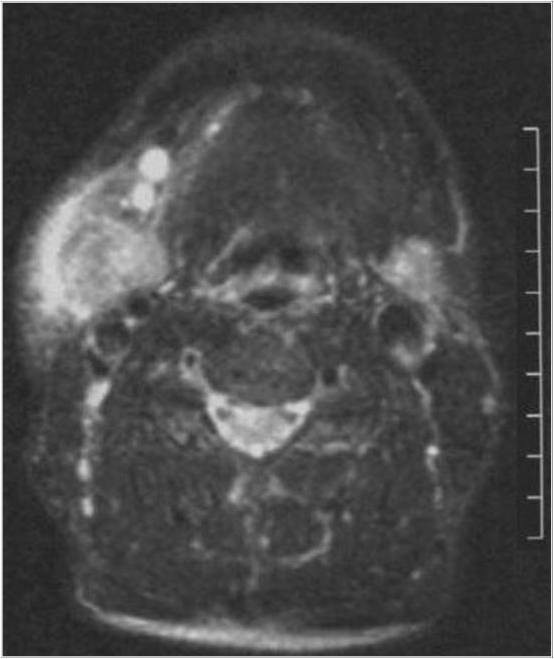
Fat-saturated T2-weighted weighted MRI. Fat-saturated T2-weighted weighted MRI showing a heterogeneous hyperintense mass with indistinct margins between the sub-mandibular gland and the lymph nodes. The mass extended invasively to the outer adjacent tissue of the sub-mandibular gland. The sub-mandibular lymph nodes were hyperintense.
